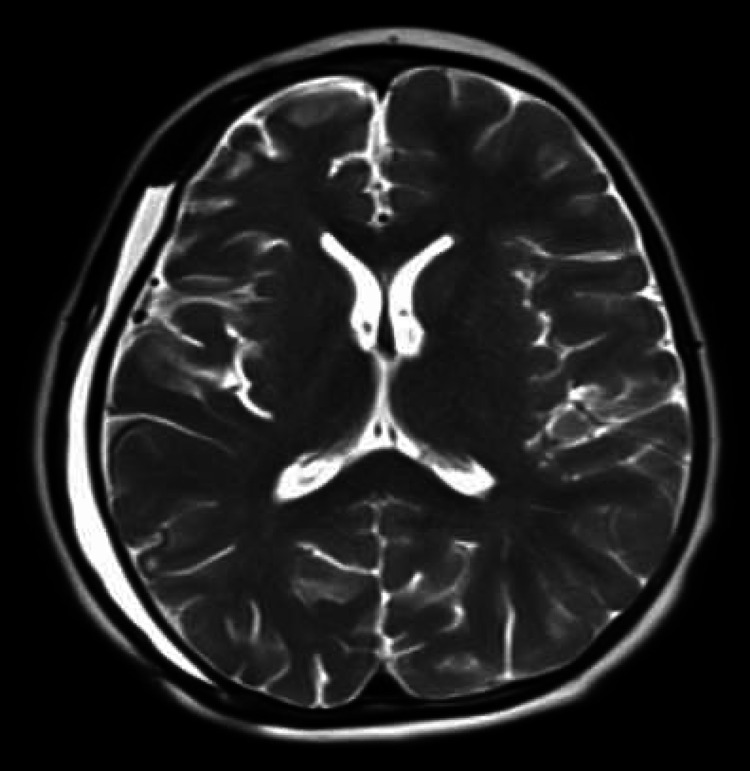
Brain MRI shunt series one month after removal of the PEEK cranioplasty Limited brain MRI shunt series one month after removal of the polyetheretherketone (PEEK) cranioplasty demonstrating resolution of the midline shift, right hemispheric compression, and columnar structures. The epidural collection is markedly reduced in size.
